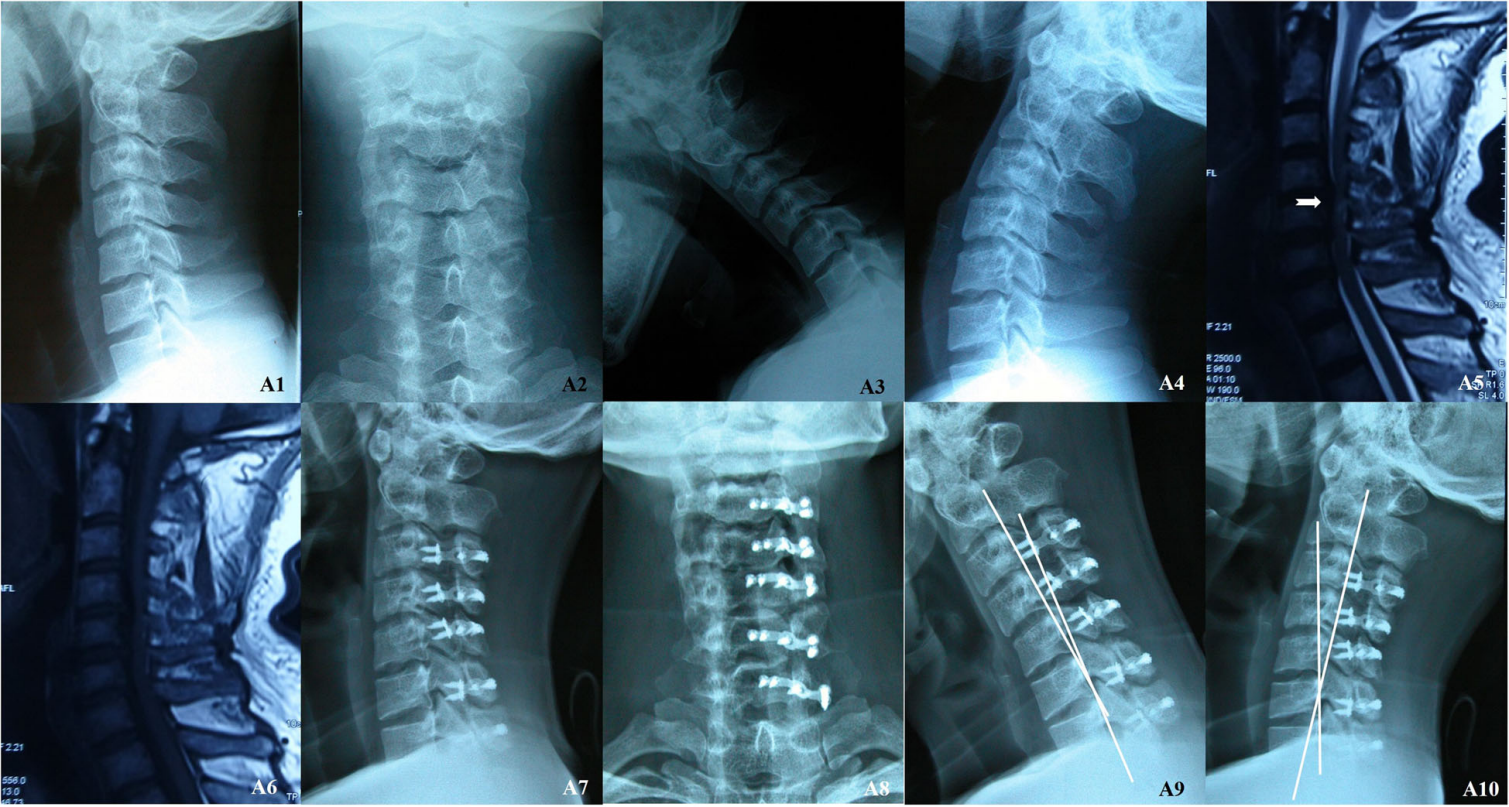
M, 42Y, multi-level cervical spondylotic myelopathy. Preoperative X-Ray images (A1 and A2); X-Ray images of extension and flexion (A3 and A4) shown no obvious cervical instability; Preoperative MRI scans (A5-A6) shown increased signal intensity on T2-weighted image (White arrow); Postoperative X-Ray images (A7 and A8); X-Ray images of extension and flexion at the 30 months after operation (A9 and A10) (White lines)
